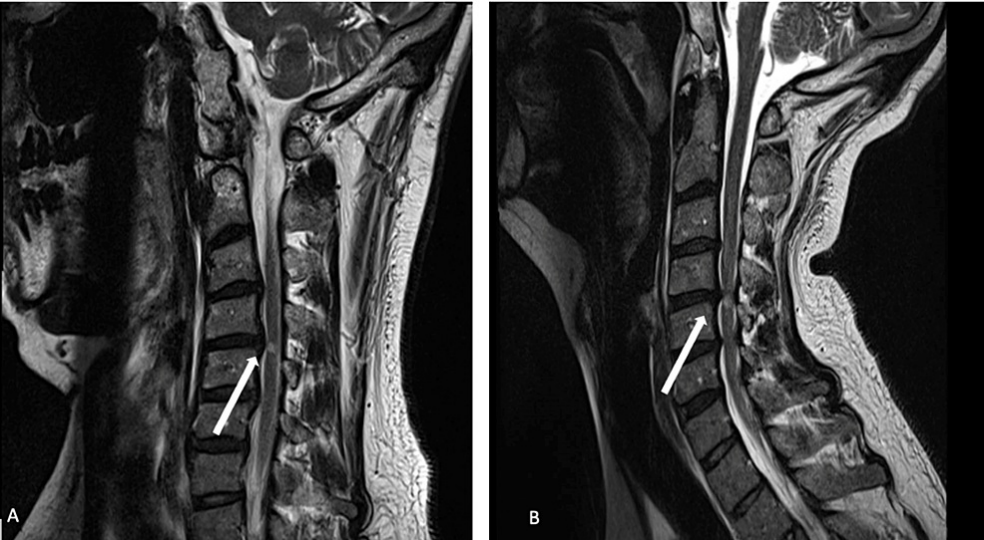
Sagittal T2-weighted MRI of the cervical spine during neck flexion (A) and neck extension (B) showing significant narrowing of the canal and effacement of the cord at the level of the signal abnormality at C4-C5 during neck extension.
